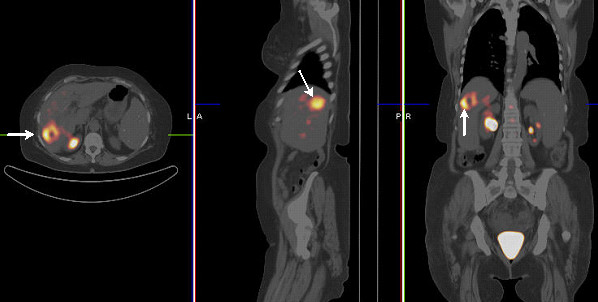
Transaxial, sagittal, and coronal PET/CT fusion images demonstrating a ring-shaped liver lesion (arrow) with increased metabolic activity (SUV_max_:5.5) surrounding a photopenic center in a patient previously treated with Yittrium-90 microspheres. CEA became positive two months after PET and subsequent pathology demonstrated tumor recurrence as well as nodal metastasis.
